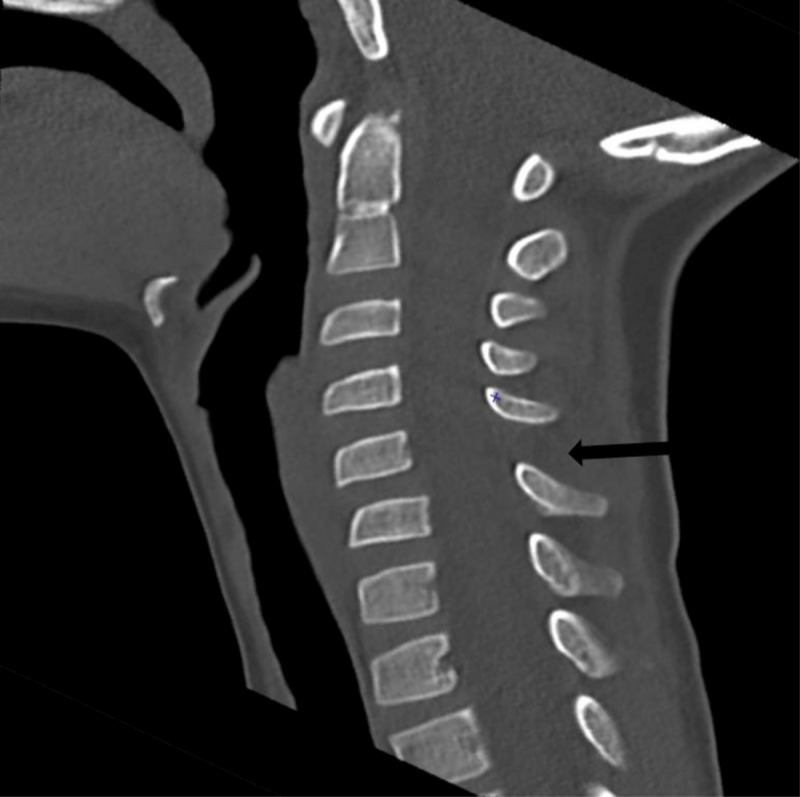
Follow-up CT exam revealing C4-C5 anterolisthesis and an increase in the corresponding interspinous space.
